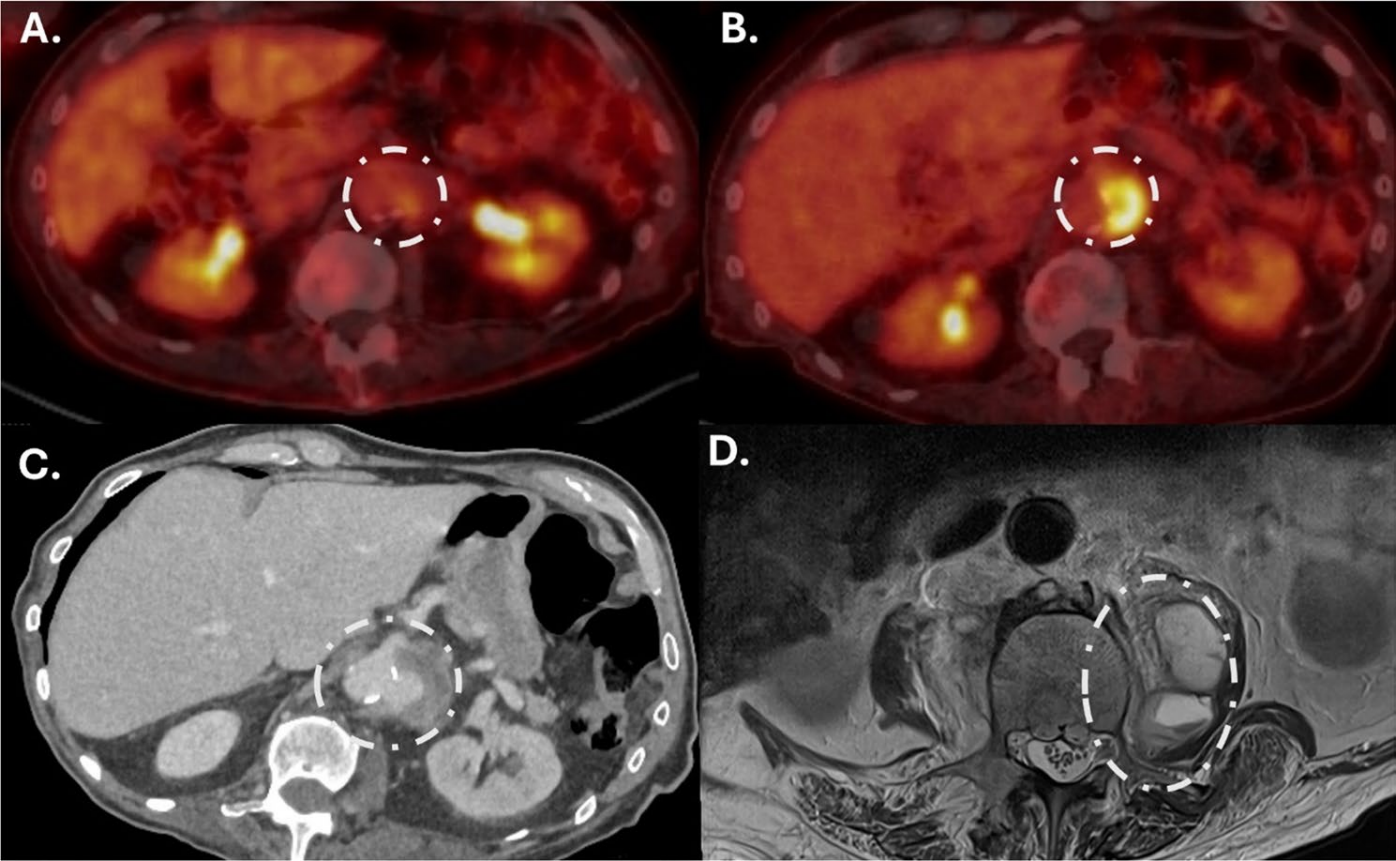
Radiological progression of BCG-associated aortic mycotic aneurysm. A Surveillance PET scan in December 2023; B surveillance PET scan June 2024 with increased avidity in the left para-aortic region; C CT-scan October 2024 with contained aortic aneurysm rupture; D MRI scan October 2024 showing left psoas abscess collection
